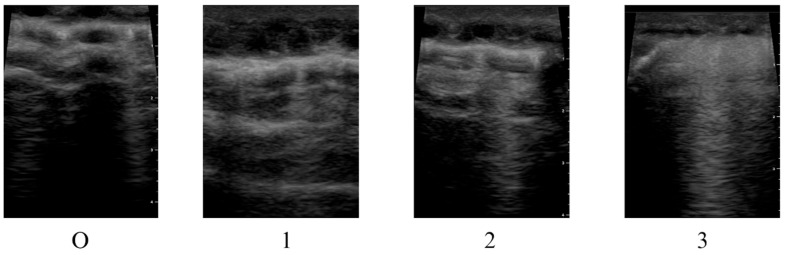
Lung ultrasound score used during study protocol. Representative ultrasound images corresponding to lung ultrasound scores: 0 = normal aeration (A-line pattern), 1 = presence of discrete B-lines, 2 = confluent B-lines or “white lung” appearance, and 3 = lung consolidation.
